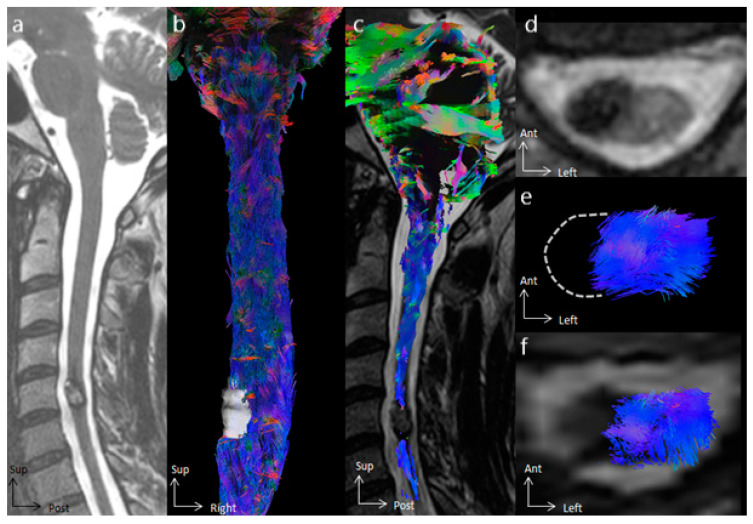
Spinal cord tractography in patient 6, with a cavernous hemangioma. (a) Sagittal T2-weighted image showing a cavernous hemangioma at the C5 spinal level, surrounded by hemosiderin crown in a hyposignal T2-weighted sequence. (b) Ventral view of the spinal cord tractography with 3D rendering of the cavernous hemangioma (in white). (c) Overlay of the spinal cord tractography on the sagittal T2-weighted MR images, demonstrating that no fibers were within the tumor. (d) Axial T2-weighted images showing a left exophytic intramedullary cavernous hemangioma. (e) Axial view of spinal cord tractography at the C5 level. (f) Overlay of the spinal cord tractography on the axial T2-weighted MR images, showing that spinal fibers were pushed back and compressed by the cavernous hemangioma, without fibers within the lesion.
